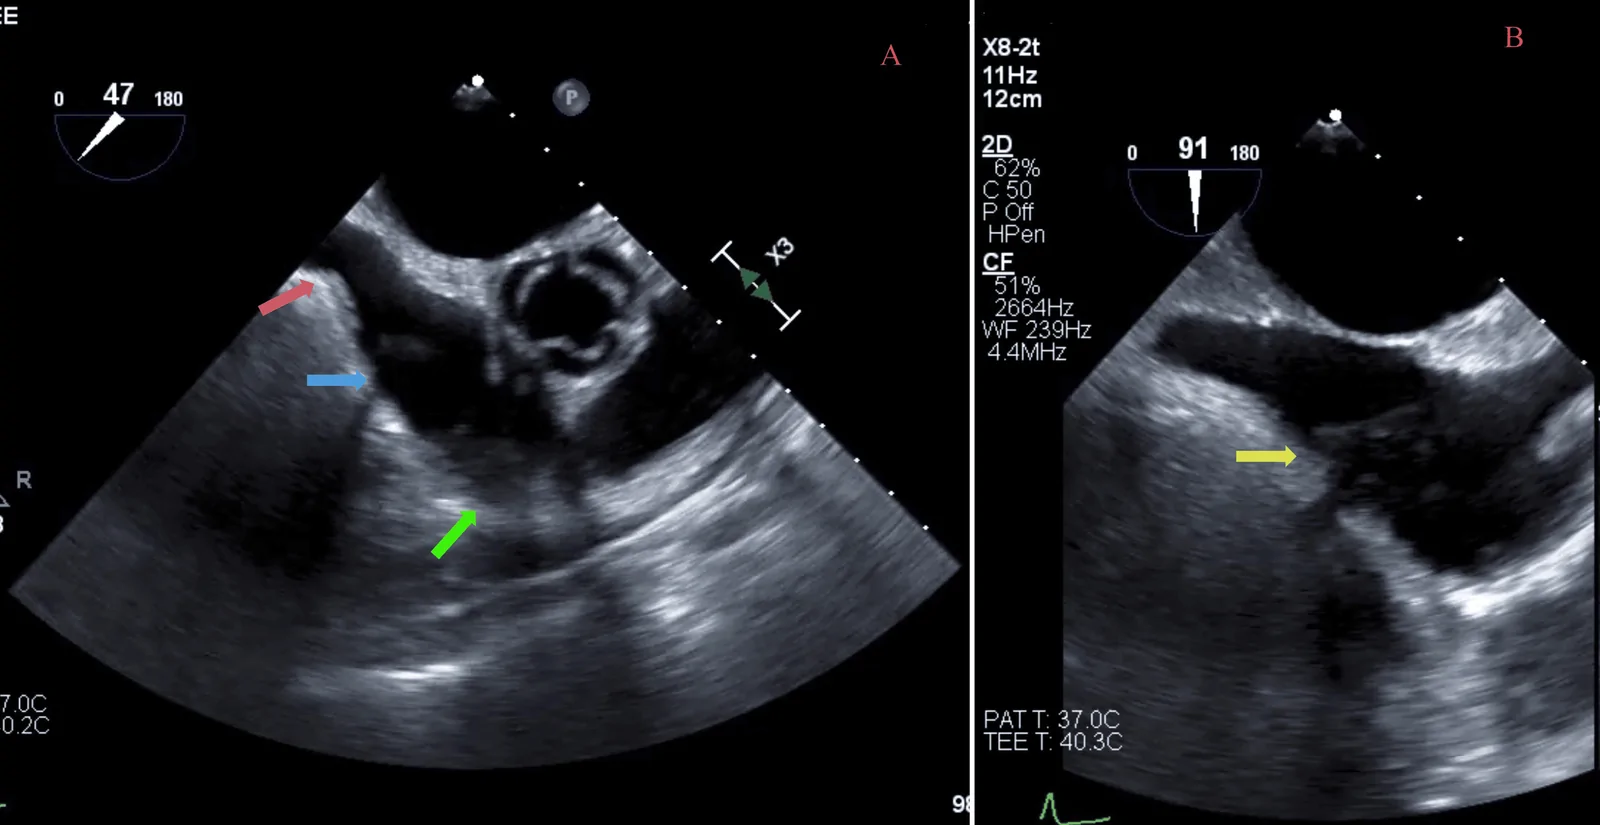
Transesophageal echocardiography. (A) Midesophageal RV inflow-outflow view demonstrating extrinsic compression of the RA-inferior vena cava junction (red arrow), RA (blue arrow), and RV free wall (green arrow). A small pericardial effusion is also present along the RV free wall. (B) Midesophageal bicaval view demonstrating RA compression (yellow arrow). RV: Right Ventricle; RA: Right Atrium
